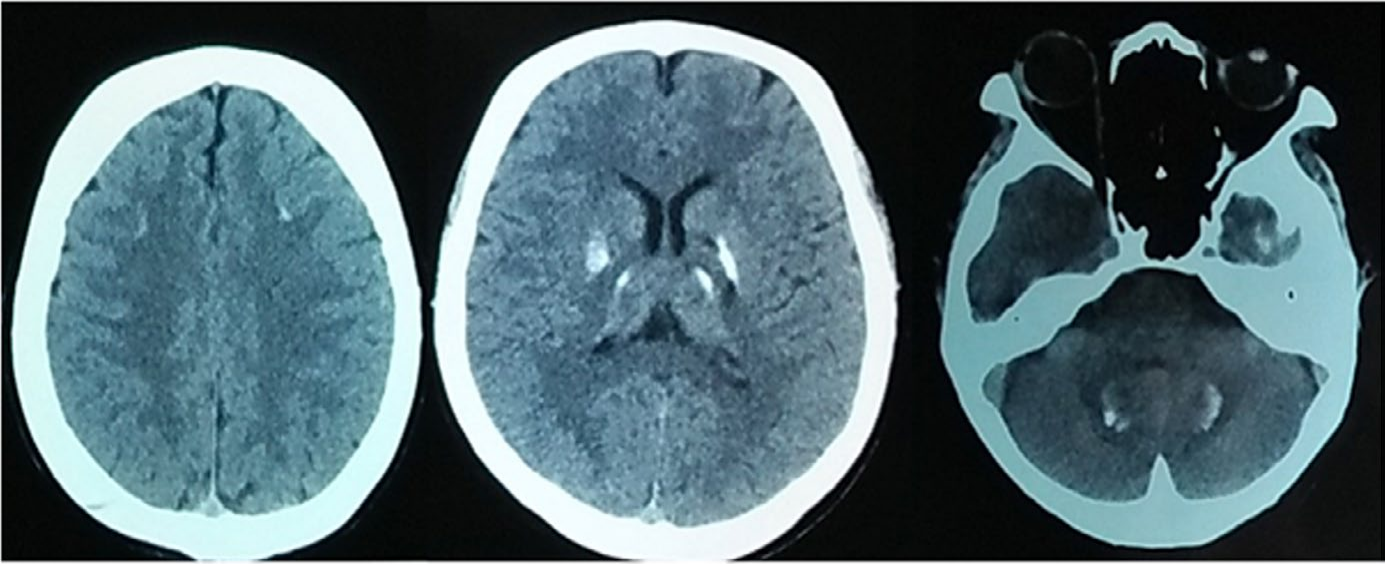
Computed tomography scan of the head showing multiple coarse calcific foci in bilateral frontal subcortical white matter, basal ganglia, thalami, and dentate nuclei
